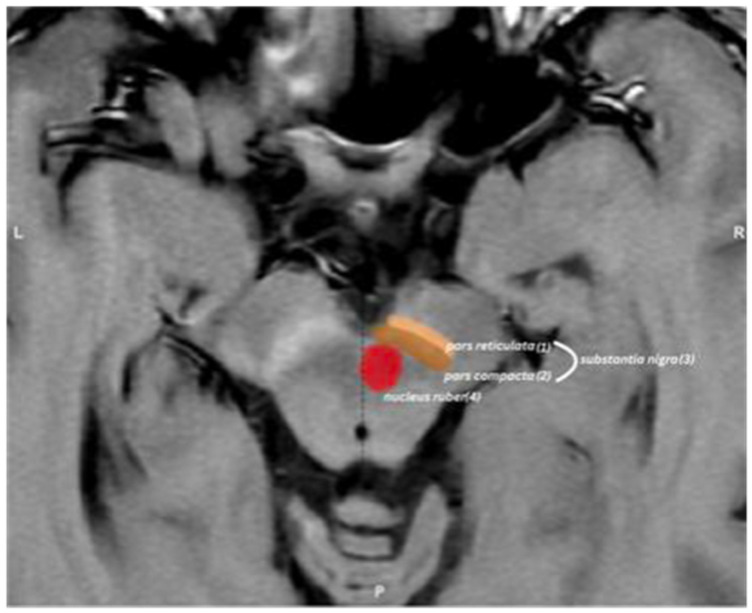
Neuromelanin visualization with NM-MRI, using adjusted T1-weighted imaging sequence: (1) Pars reticulata; (2) Pars compacta; (3) Substantia nigra; (4) Nucleus ruber.
